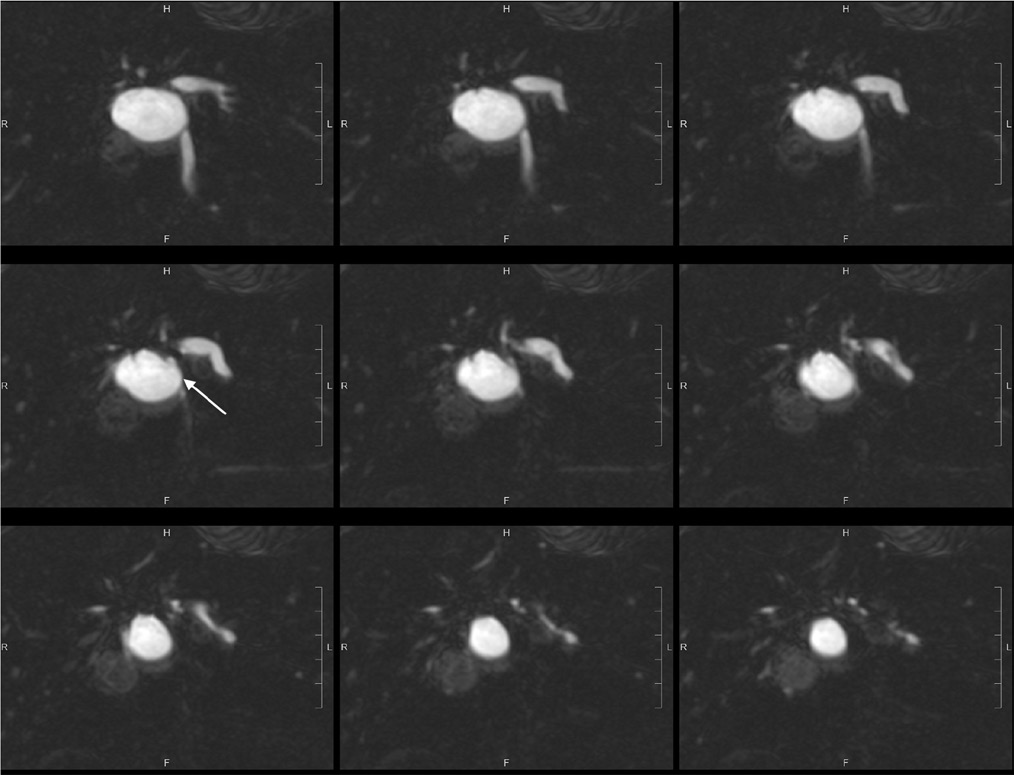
Post-oral contrast T2-weighted coronal MRCP images (SPACE 1mm with reformat)
